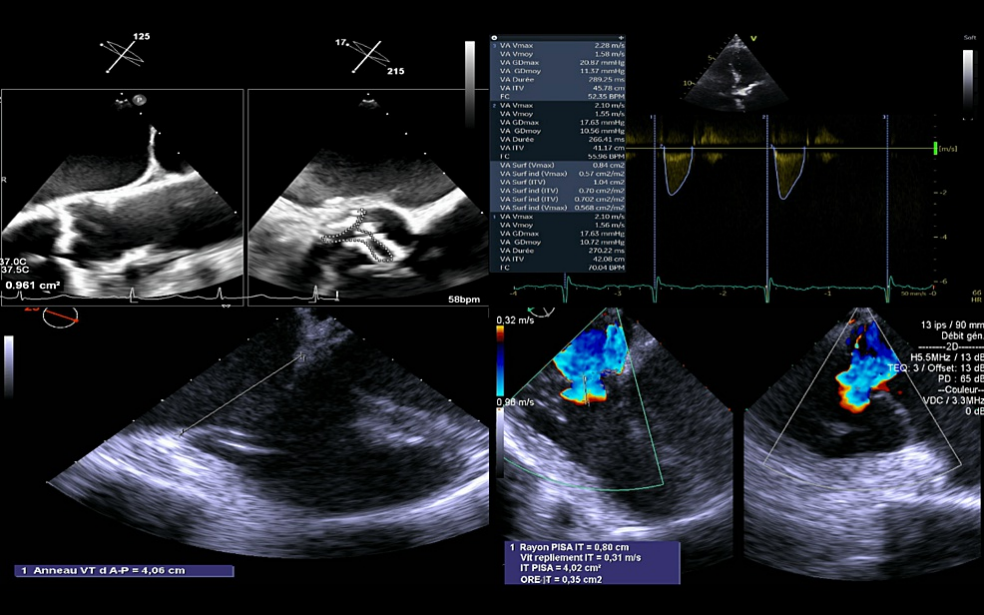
Preoperative echocardiogram images showing severe AS and severe TR AS: aortic valve stenosis; TR: tricuspid valve regurgitation
